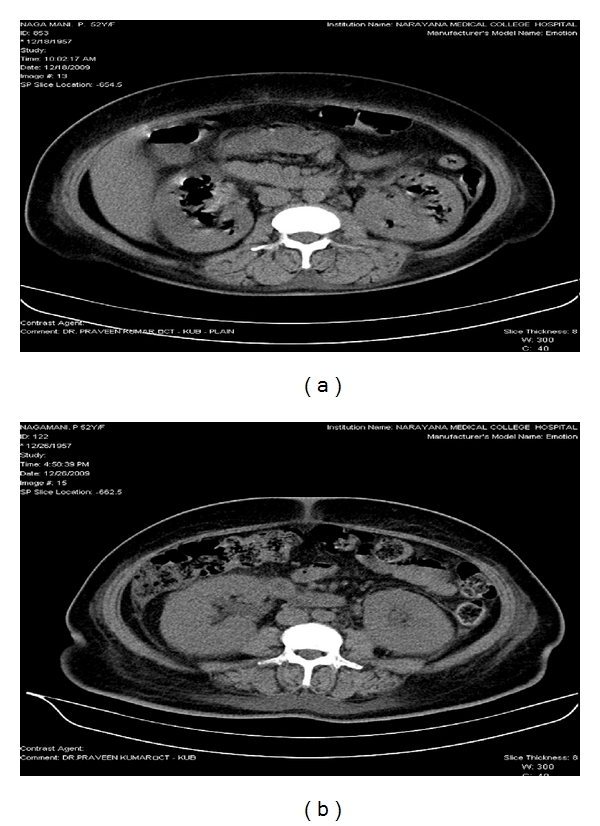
(a) CT KUB showing bilateral emphysematous pyelonephritis (Class IV). (b) CT KUB showing resolution of air pockets after 10 days of treatment.
